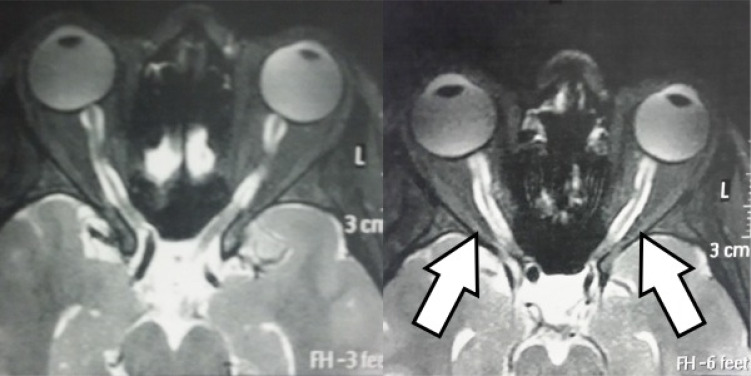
Orbital resonance showing bilateral longitudinally extensive optic neuritis. Axial section with T2-weighted acquisition.
